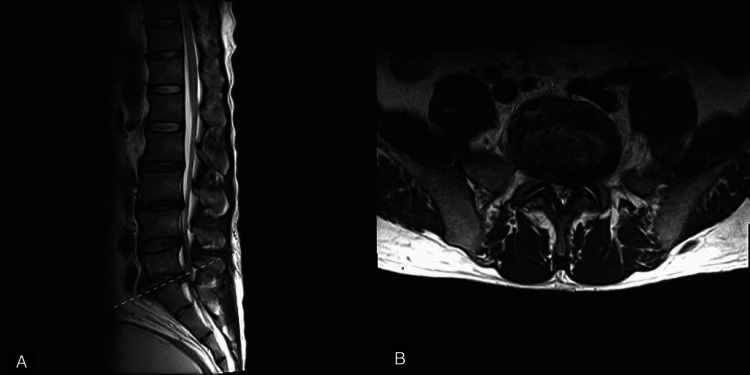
MRI of the lumbosacral spine. (A) The sagittal image shows irregular changes in the end plate with fluid accumulation at the L5/S1 disc space, suggestive of spondylodiscitis. Additionally, disc protrusions are noted at L3/4, L4/5, and L5/S1, along with spinal stenosis, which are most pronounced at L5/S1; (B) The axial view at the L5/S1 level (dashed line in A) reveals a disc protrusion with spinal stenosis, more prominent on the left side.
